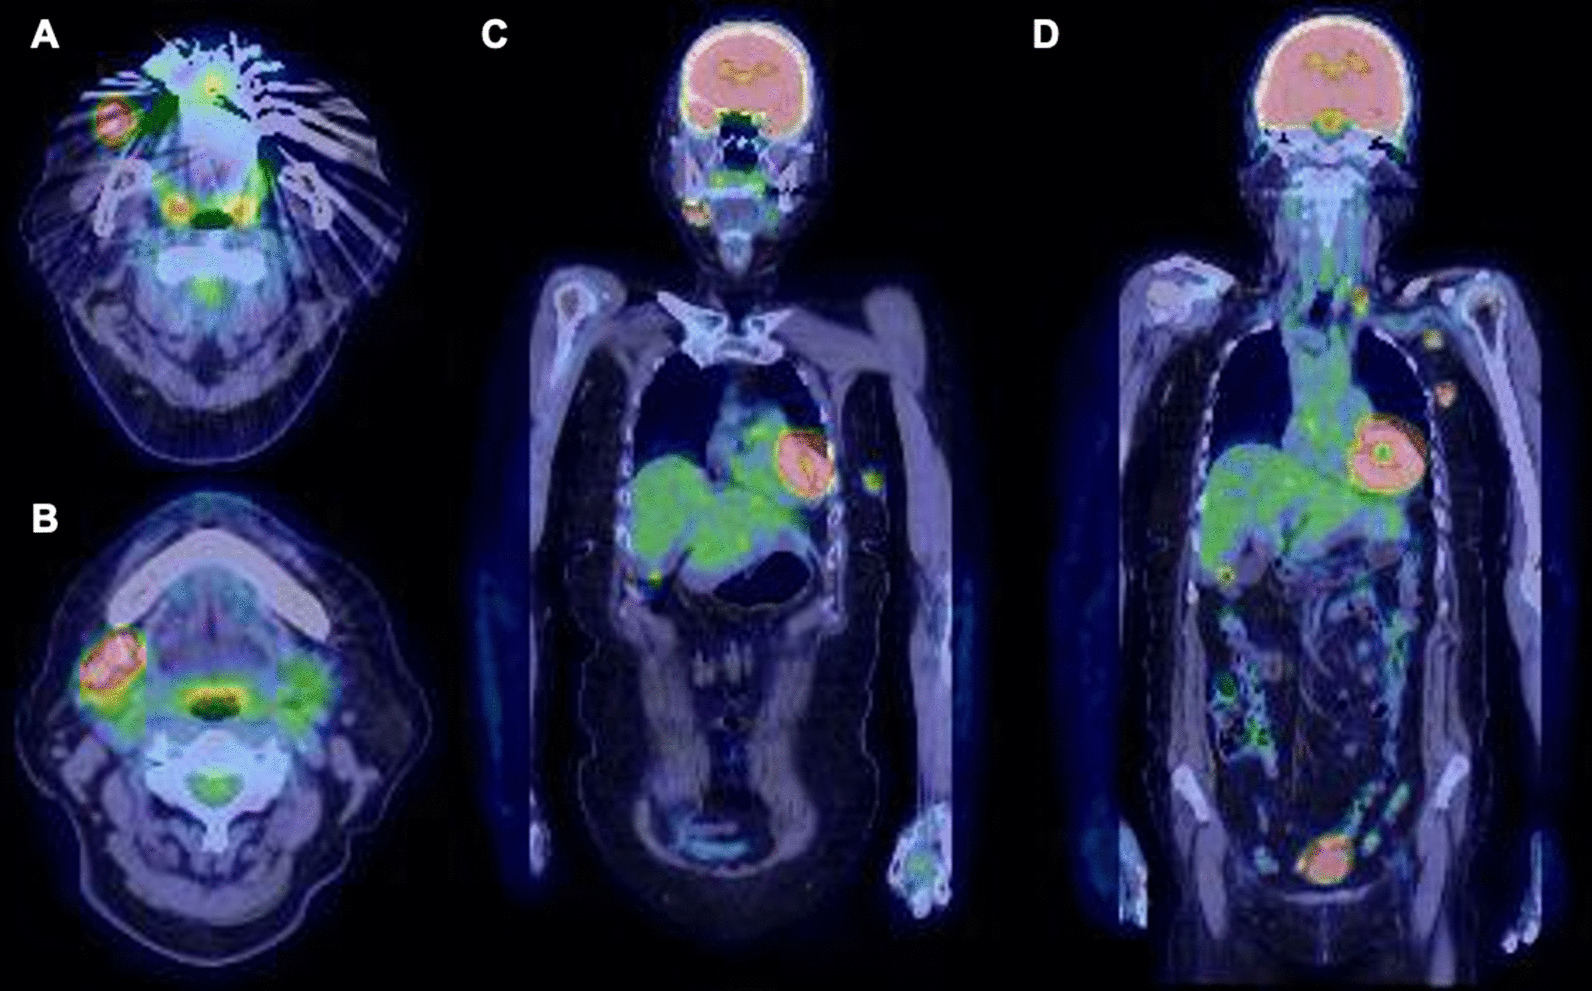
FDG-PET/CT showed high signals in the right buccal region (A), right submandibular lymph node (B), left breast (C), and lymph nodes in the left supraclavicular fossa and axilla (D)
